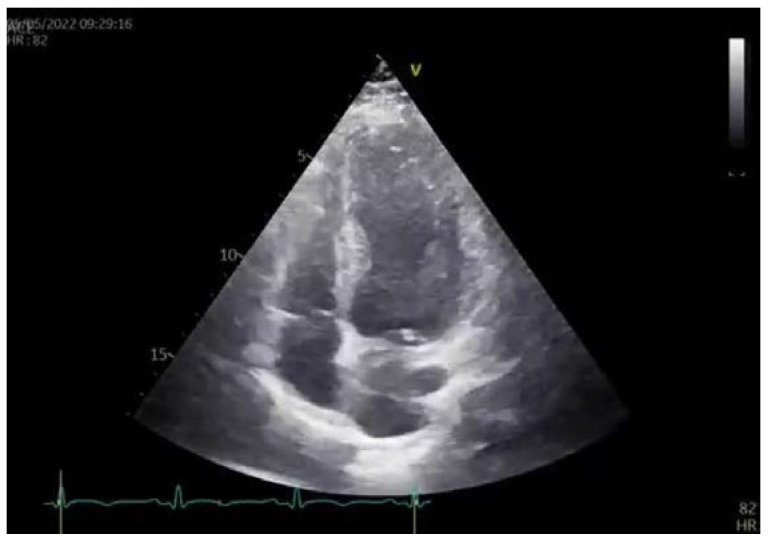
Transthoracic 2D echocardiography showed akinesia of the apical segments of all walls and there was akinesia in practically one-half of the left ventricle (LV). However, the thicknesses of akinetic walls were preserved. Left ventricle ejection fraction (LVEF) was 39%. The echocardiography exam is presented in Videos S3 and S4. Global longitudinal strain (GLS) was −9.7%. Apical segments of LV, assessed by 2D strain echocardiography, were blue-colored in the bull’s eye plot, which was a sign that these segments had completely impaired strain movements, while medial segments of LV had reduced strain movements. Basal segments of LV had good strain movement. Several routine blood and biochemical tests were conducted which showed the following values of two peaks of cardio-specific enzymes on the second and fifth days. Hormonal thyroid status was normal.
